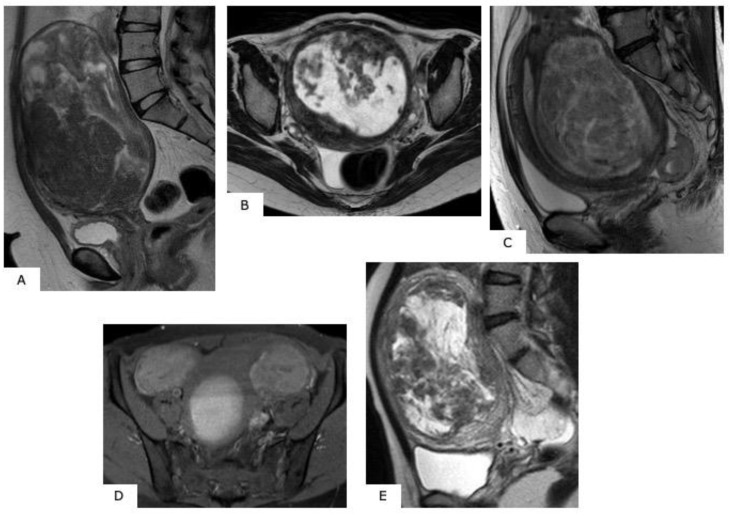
MR images of degenerated leiomyoma. These images always require a differential diagnosis from sarcoma. (A): hyaline degeneration (T2WI), (B): leiomyoma with cystic change (T2WI), (C): cellular leiomyoma (T2WI), (D): red degeneration (T1WI), (E): myxoid degeneration (T2WI).
